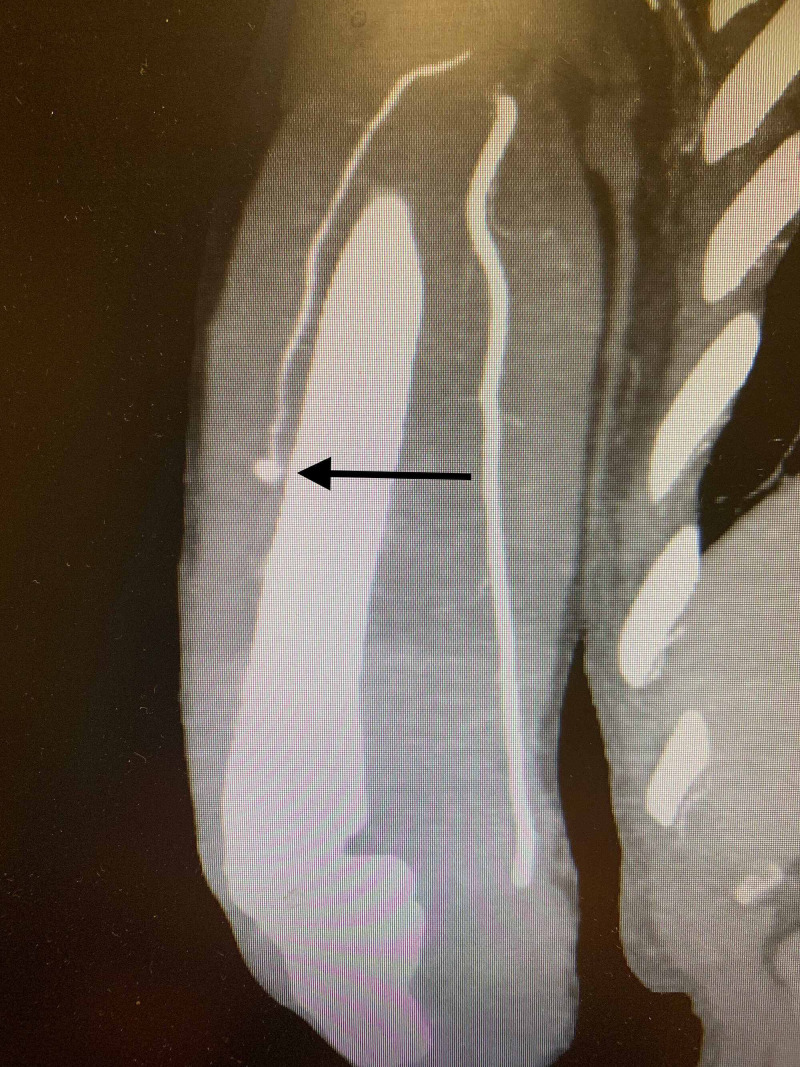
CTA of the right upper extremity demonstrating active bleed CTA: computed tomography angiogram
